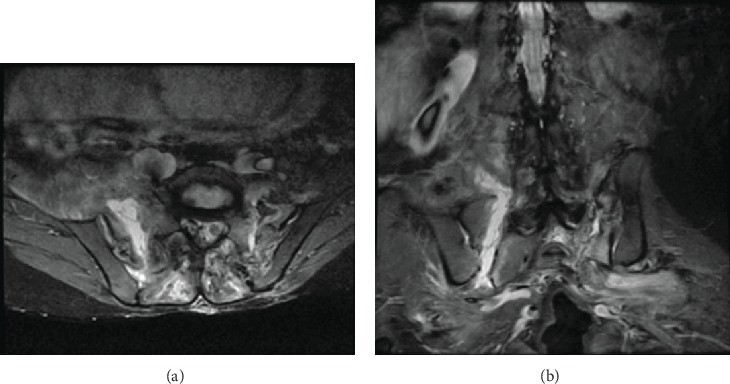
MRI showed an extensive oedema of the posterior paraspinal muscles bilaterally from L4 to S2 levels and diffuse marrow oedema of the sacrum. A large collection in the right posterior paraspinal muscles tracking inferiorly and anteriorly through a large bony defect in the right half of the sacrum seen in the axial (a) and coronal (b) views. There are bilateral spinal-pelvic fractures and dissociation.
